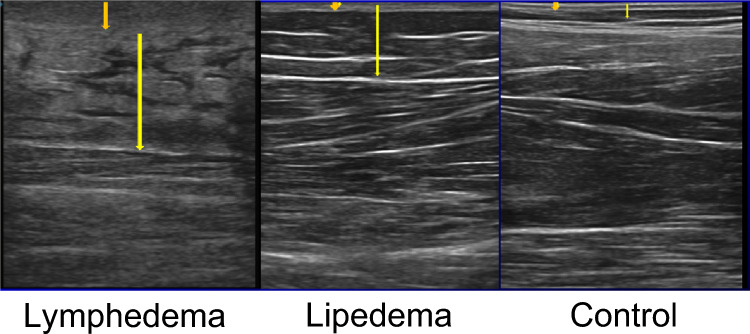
Ultrasound to distinguish normal, lymphedema and lipedema. Lymphedema is associated with increased dermal thickness and with subcutaneous tissue hyperechogenicity, whereas lipedema is associated with increased thickness and hypoechogenicity of the subcutaneous fat (dermis marked in upper arrows, subcutaneous tissues in lower arrows)
